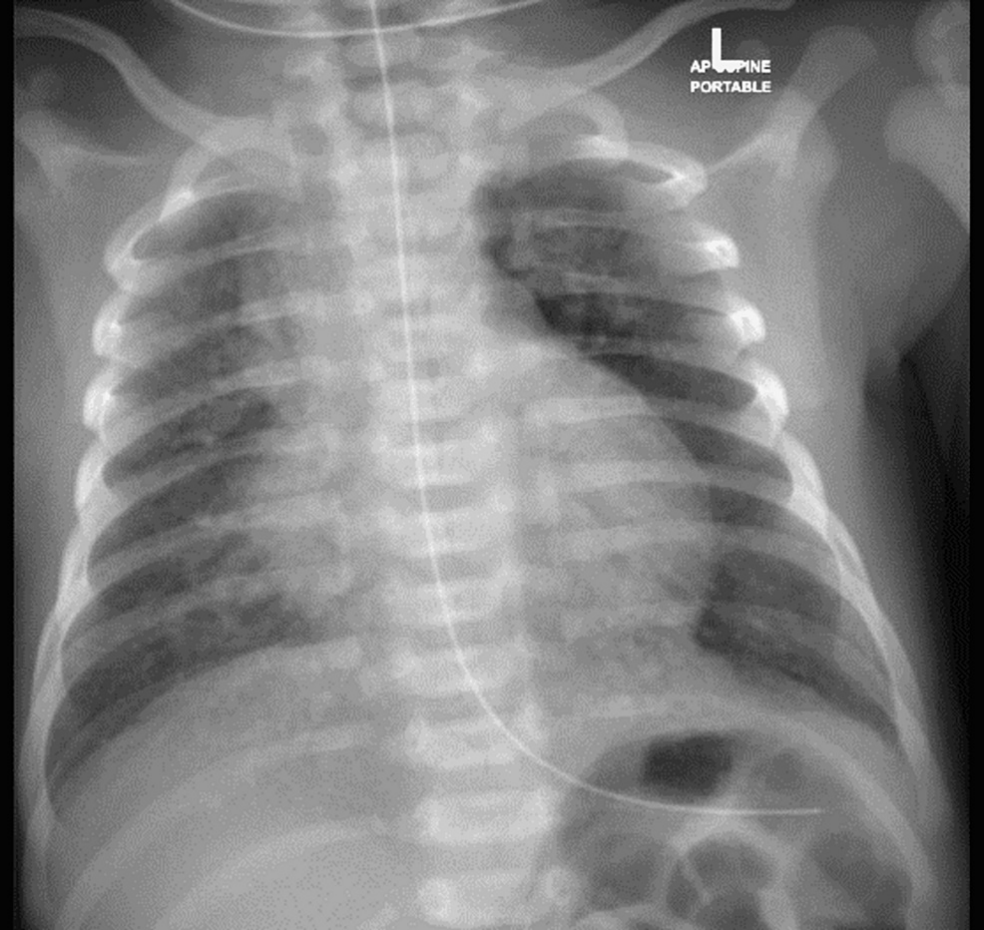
First chest X-ray before LISA LISA: Less invasive surfactant administration
